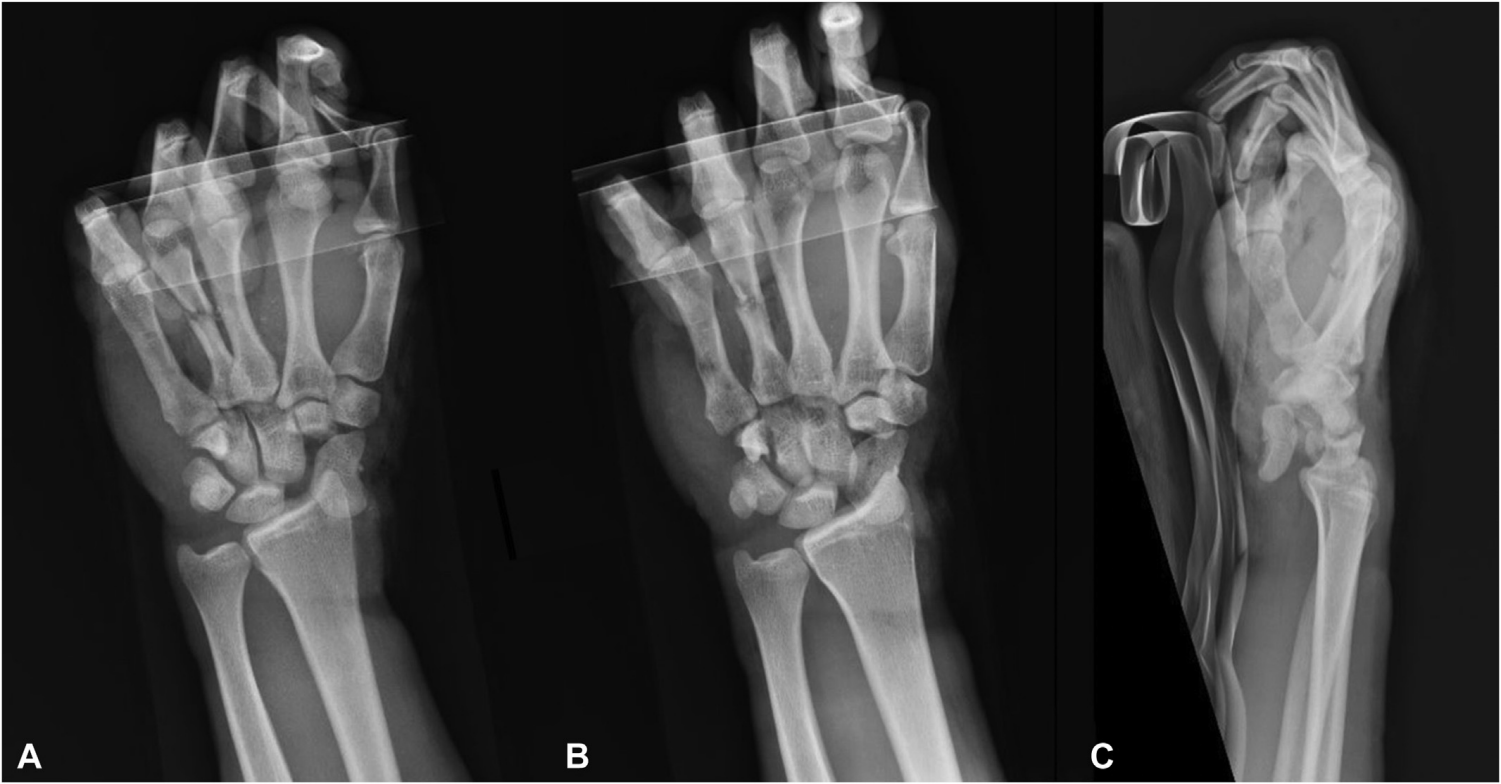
Three radiographic views of the left wrist. A Posterior-anterior view. B Oblique view. C Lateral view. These images demonstrate a palmar–radial scaphoid dislocation, a hamate body fracture at the base of the hook of hamate, and a fourth metacarpal midshaft fracture.
